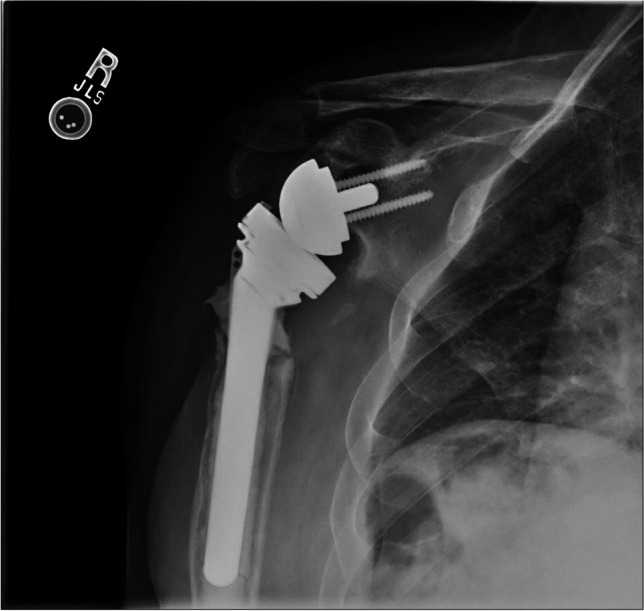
Radiographs of patient 3 years after reverse total shoulder arthroplasty with circumferential radiolucency between bone-cement interface, cortical erosion, and distal cement fracture concerning infection
